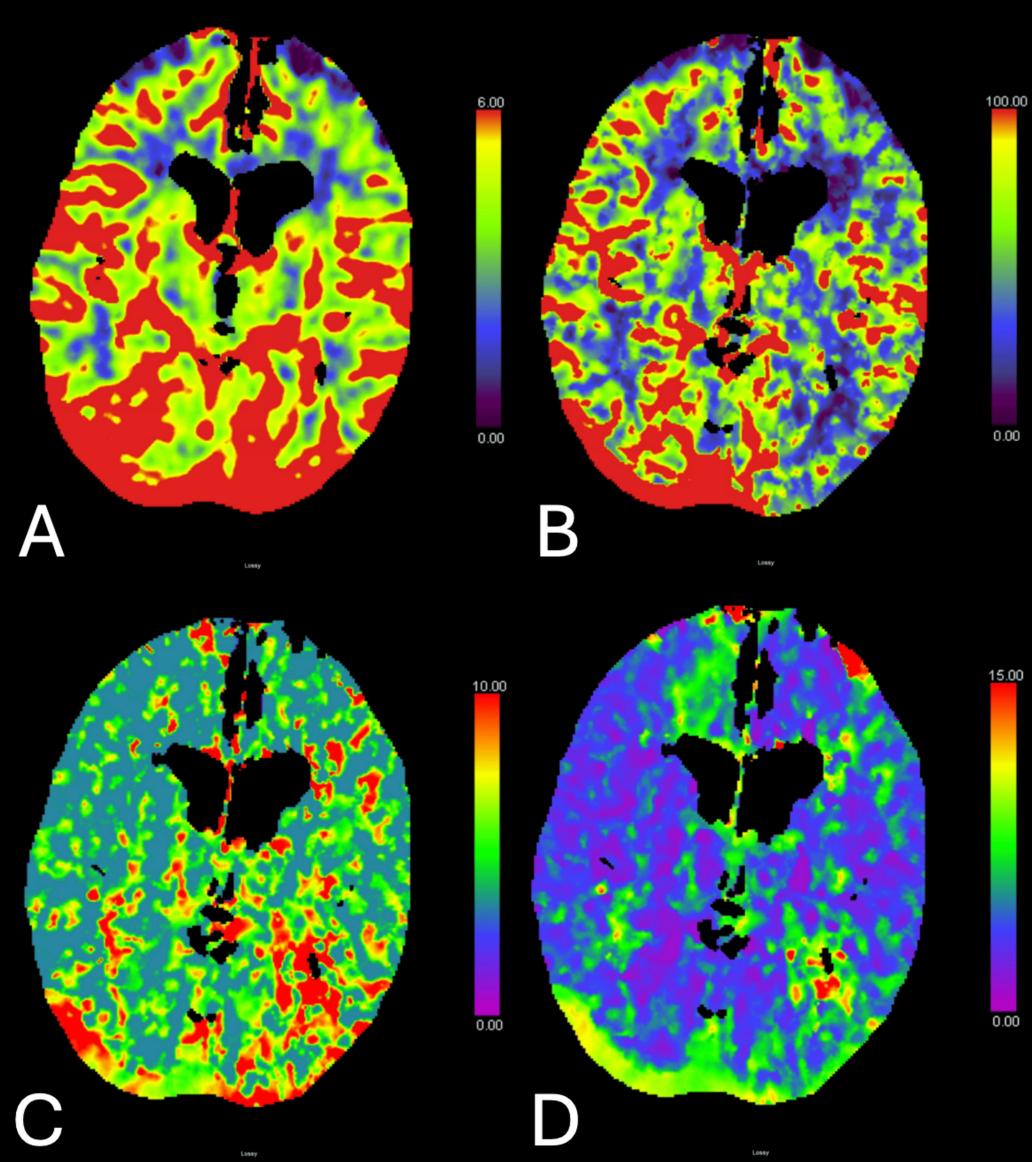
Brain CT perfusion (CTP) with contrast, axial view. CTP taken during admission. (A) Cerebral blood volume: increased on the right parietal lobe, appearing as red areas indicating higher blood volume. (B) Cerebral blood flow: increased on the right parietal lobe, shown as red areas corresponding to higher blood flow. (C) Mean transit time: decreased on the right parietal lobe, with blue areas representing shorter perfusion times. (D) Time to drain: decreased on the right parietal lobe, shown as blue areas indicating faster venous outflow.
